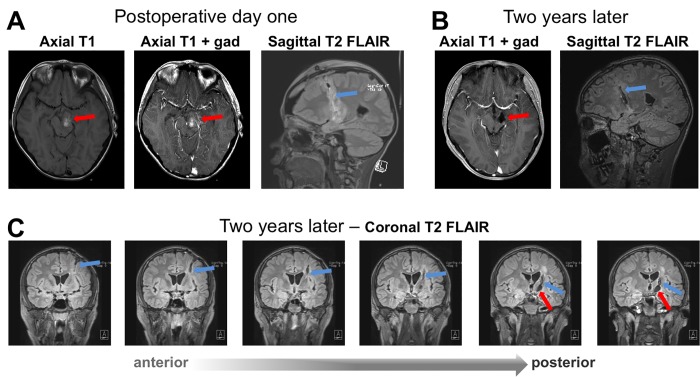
Postoperative magnetic resonance imaging (MRI). Postoperative MRIs obtained on day one (A) and two years (B and C) after surgery demonstrate gross total resection of the juvenile pilocytic astrocytoma (JPA) and no recurrence. The panel in (C) represents serial thin-cut 1 mm T2 fluid-attenuated inversion recovery (FLAIR) images on the coronal plane that capture the surgical corridor two years after the procedure. Note: The trajectory traverses the anterior limb of the internal capsule as planned and is surrounded by only small amounts of FLAIR hyperintensity. This suggests a limited gliosis due to injury during the approach. The red arrow: the surgical cavity; blue arrow: trajectory.
